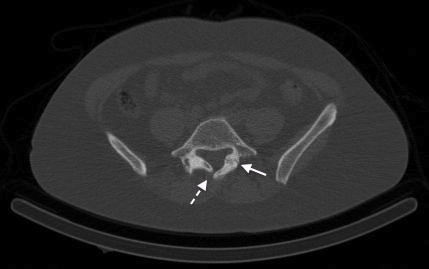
CT image from SPECT/CT demonstrating discontinuity of the left pars interarticularis (continuous arrow) and an incidental spina bifida occulta (dashed arrow).
